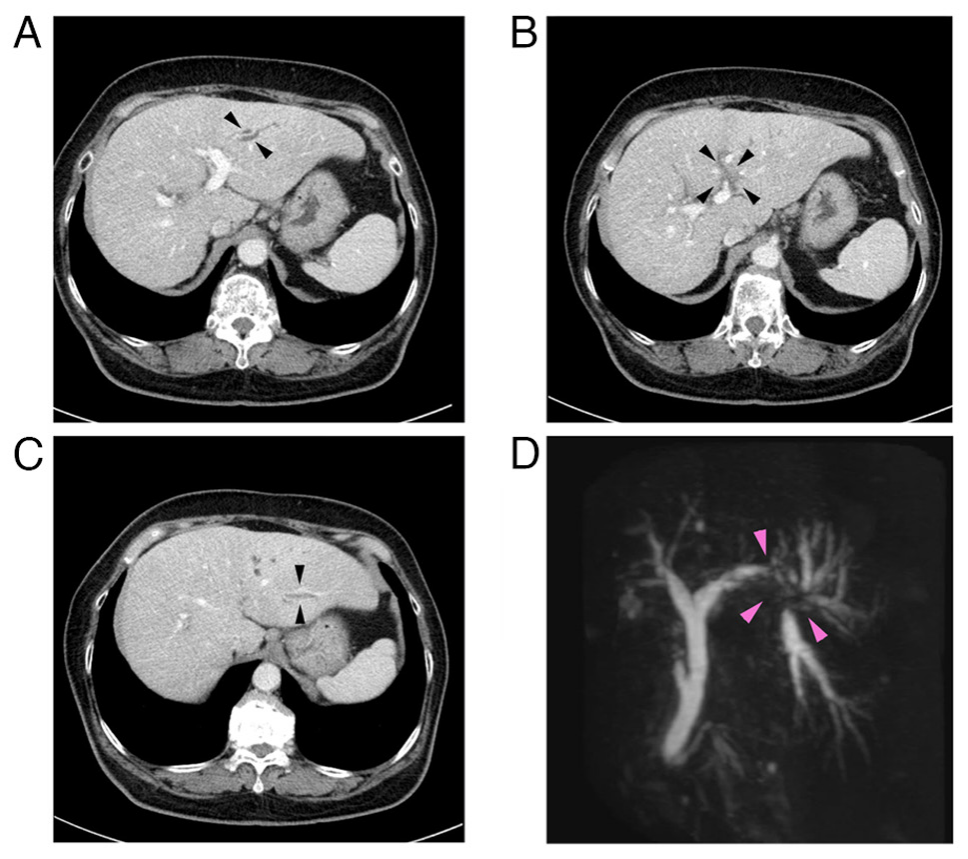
Imaging studies. (A) CECT in the portal phase demonstrated the dilatation of the B3 bile duct. (B) CECT in the portal phase demonstrated a 1x1 cm-sized low density lesion adjacent to the umbilical portion of the left portal vein. (C) CECT in the portal phase demonstrated dilatation of the B2 bile duct. (D) Magnetic resonance cholangiopancreatography revealed stricture and separation of the left branch of intrahepatic bile duct at the bifurcation of B2 and B3 bile ducts (arrows). CECT, contrast-enhanced computed tomography.
